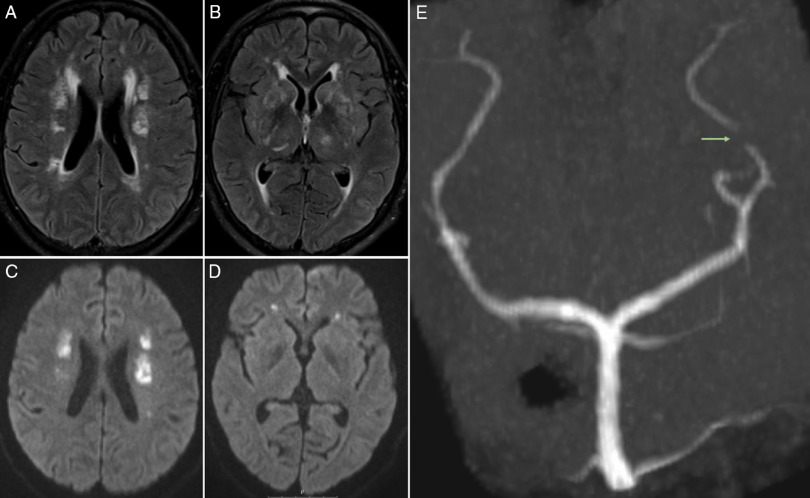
(A, B) Axial fluid-attenuated inversion recovery (FLAIR) sequence depicts multiple hyperintense lesions involving the deep and subcortical white matter on both hemispheres, as well as the thalami and basal ganglia; some of the lesions showed restricted diffusion on diffusion-weighted imaging (DWI) (C, D), namely on both corona radiata and bilateral deep frontal white matter. (E) Three-dimensional time-of-flight (3D TOF) sequence, Maximum intensity projection (MIP) reconstruction on coronal view reveals prominent irregularity (arrow) on the P3 segment of the left posterior cerebral artery.
